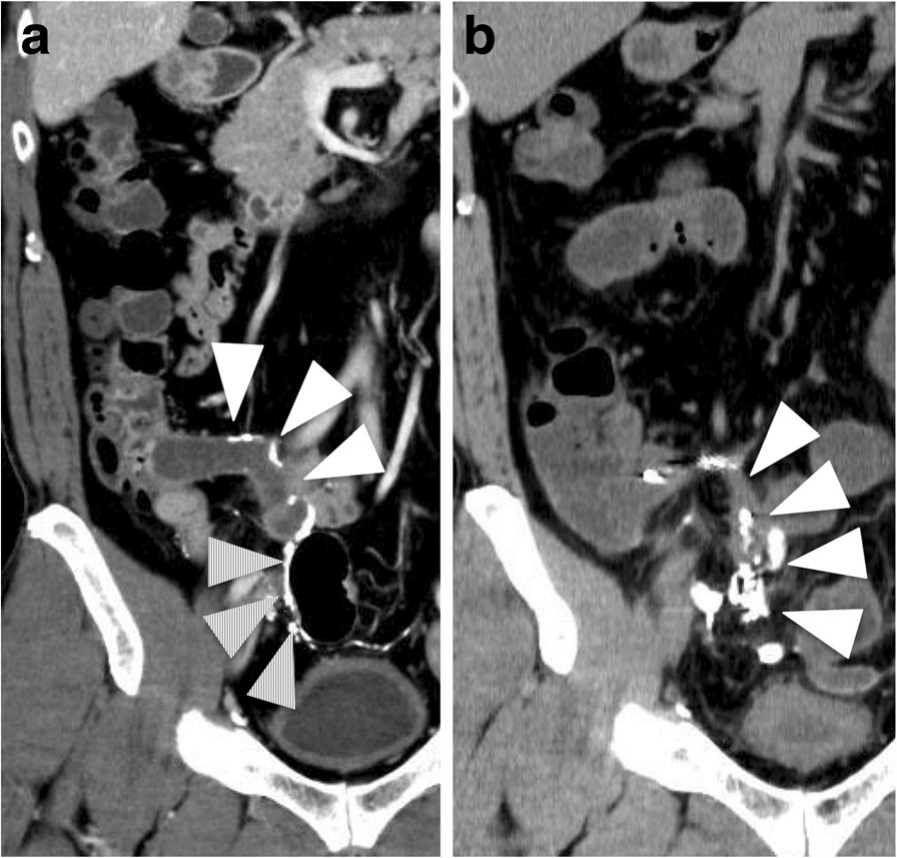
a Abdominal CT revealed a cystic lesion, measuring 10 × 3 cm, in the appendix and barium around the appendix (white triangle), and sigmoid colon (triangle with diagonal lines). b At the time of perforation of the appendix about 3 years ago, there was no finding of AMN, and barium leaked from the tip of the appendix
